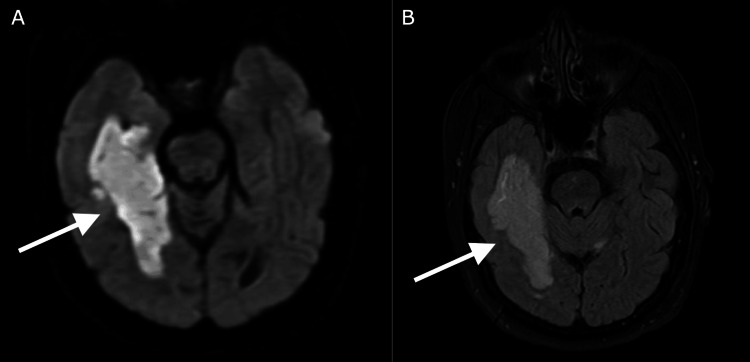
MRI brain with arrows indicating a right posterior cerebral artery territory infarct with (A) DWI hyperintensity and (B) corresponding T2 FLAIR sequencing hyperintensity. DWI: diffusion-weighted imaging; FLAIR: fluid-attenuated inversion recovery
